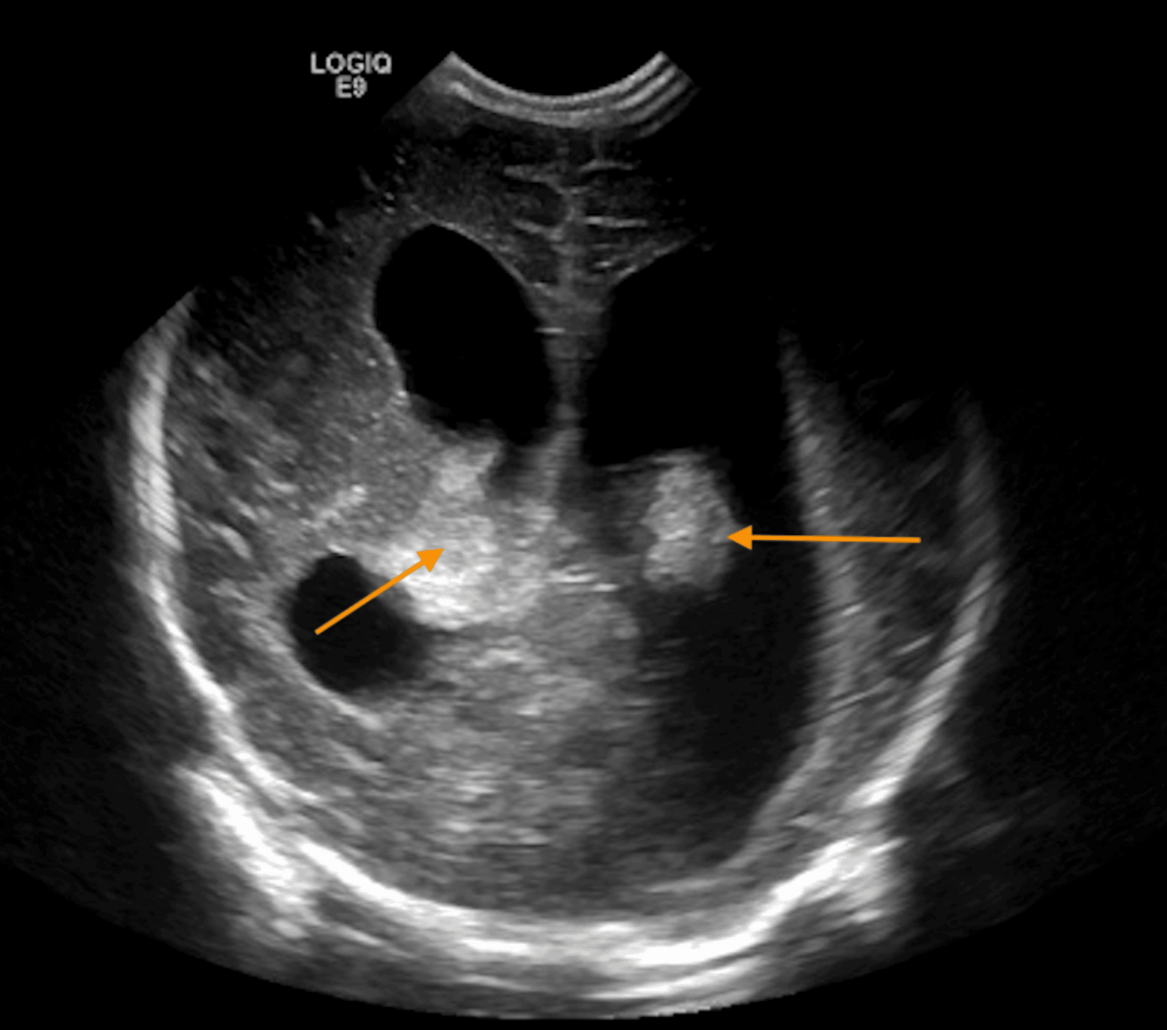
Coronal cranial ultrasound image (Case 2) demonstrating a grade 3 intraventricular hemorrhage and ventriculomegaly. Arrows indicate echogenic material within the dilated lateral ventricles, consistent with intraventricular blood.
